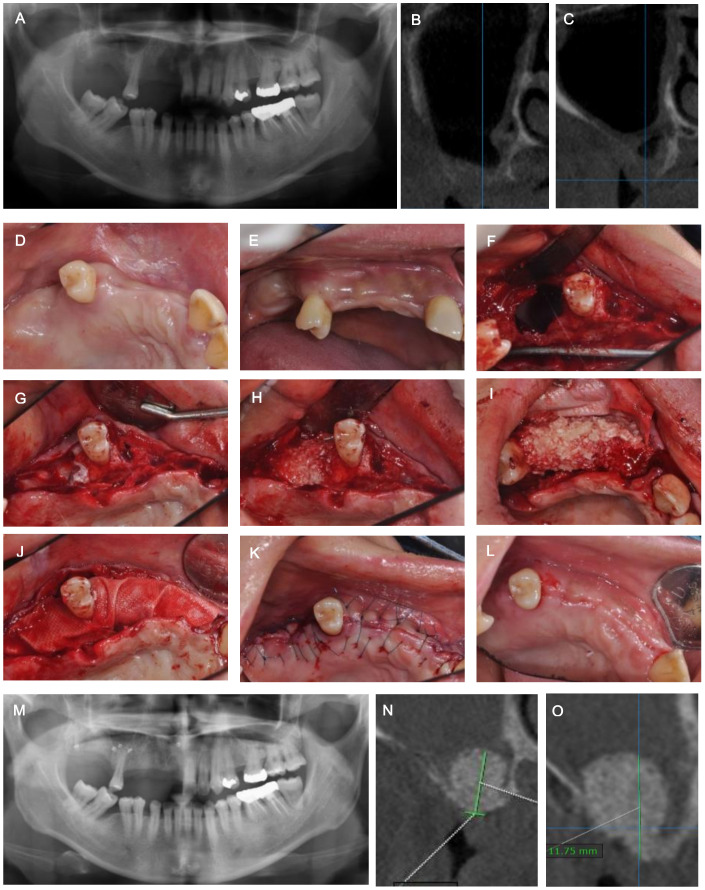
(A) Pre-operative panoramic view. (B) Pre-operative CT view of #17. (C) Pre-operative CT view of #16. (D,E) Pre-operative clinical view. (F) Oro-antral communication (OAC). (G) Collagen membrane application in sinus achieved by making pouch shape. (H) Sinus bone graft with allogenic bone material. (I) Horizontal ridge augmentation of #11-14 area. (J) Collagen membrane application on the crestal area. (K) Suturing. (L) Healing condition at 2 weeks postoperatively. (M) Panoramic view after OAC closure and bone augmentation. (N) Post-operative CT view of #17. (O) Post-operative CT view of #16.
